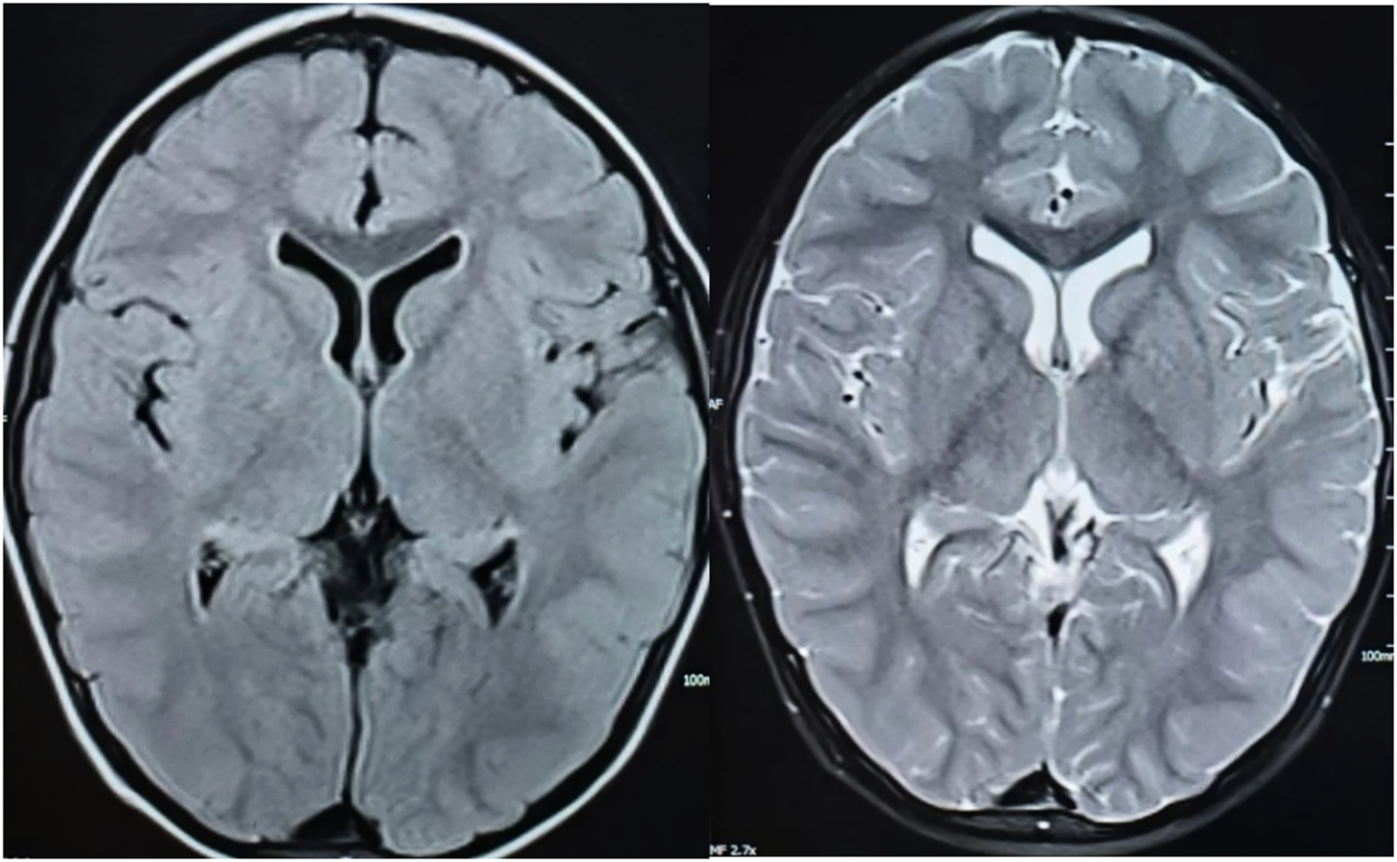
T1 (left) and T2 (right) weighted images of the child's cranial MRI scanning transverse position.
